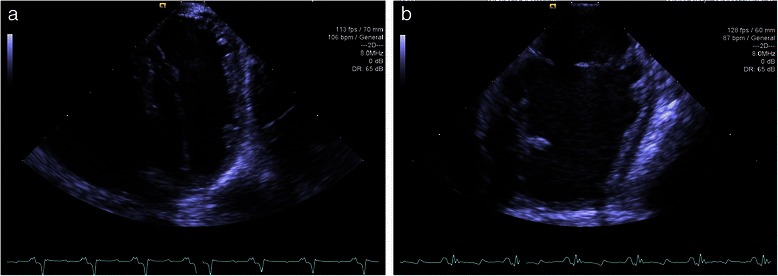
Representative intracardiac echocardiogram (ICE) images of the right ventricle (RV) at end-expiration and end-diastole. (a) PEEP 0 and (b) PEEP 30. Imaging optimized to assess the RV free wall, including the tricuspid annulus throughout the cardiac cycle, maximizing for frame rate to allow for accurate speckle tracking assessment.
